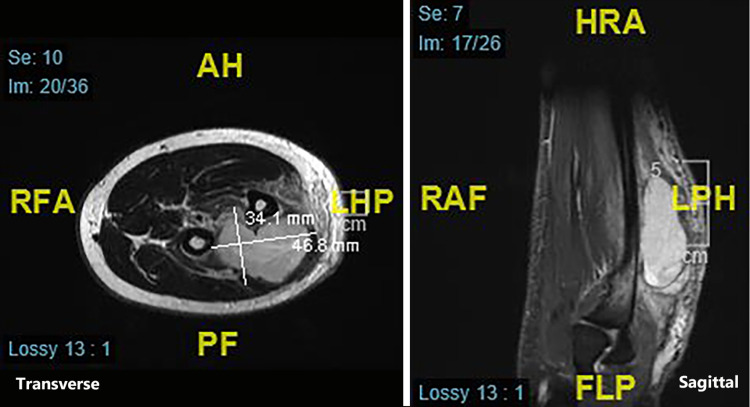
Repeat MRI scan with significant progression of the malignant peripheral nerve sheath tumour (MPNST) evident
